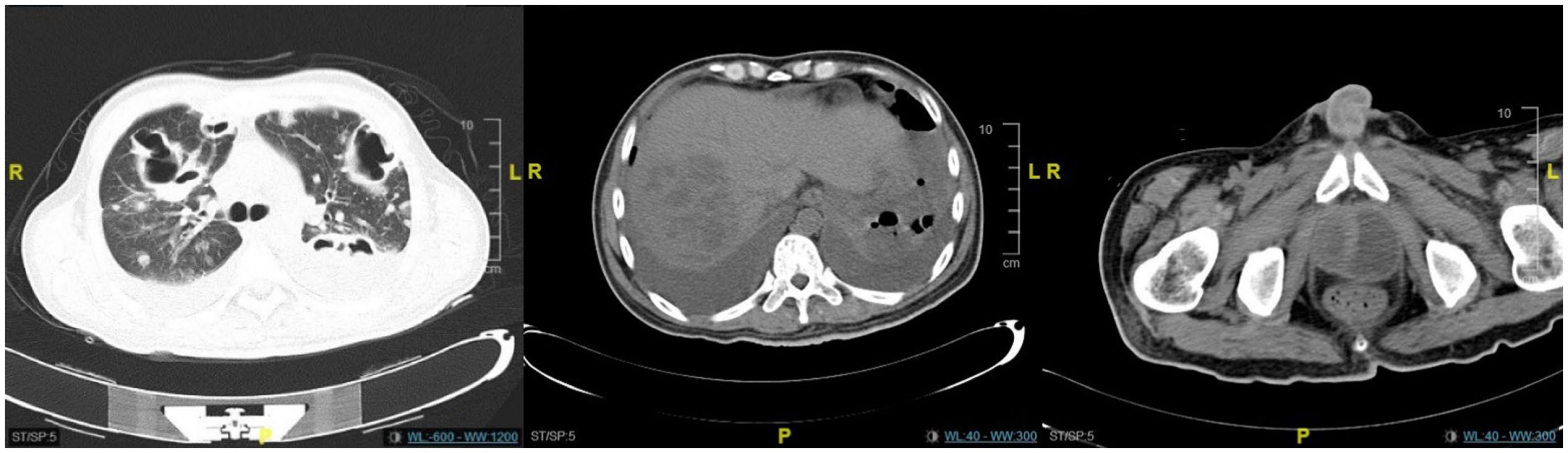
The CT scan revealed no significant improvement in the lung lesions, liver abscess, or prostate lesions.
